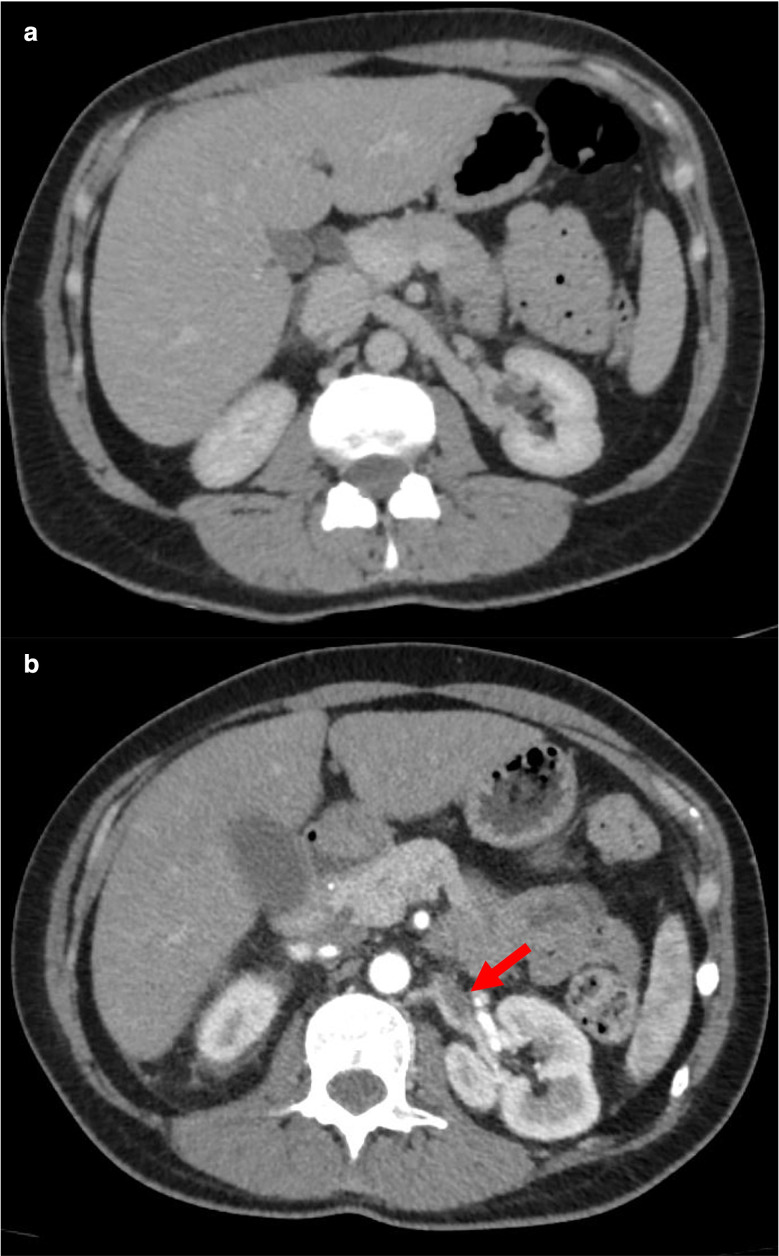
(A) Initial CT of the abdomen arterial phase on day of admission showing normal opacification of the renal arteries. (B) CT of the abdomen arterial phase showing a subocclusive filling defect in the left renal vein (arrow) with a well opacified renal artery Day 5 post-admission.
